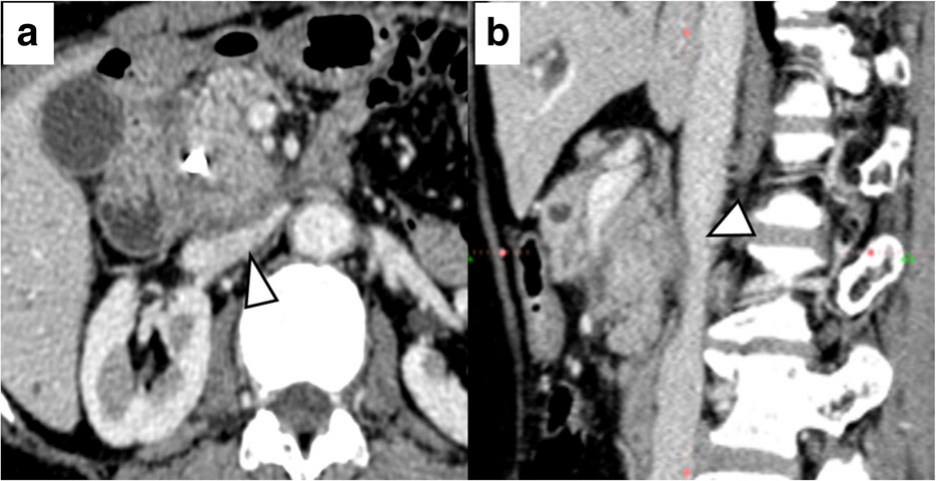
Preoperative computed tomography (CT) imaging of case 3. a Dense soft tissue connected to the pancreatic lesion widely contacting the ventral surface of the inferior vena cava (IVC) (*arrowhead*). b. Sagittal multi-planar reformation showed that the lesion adhered to and deformed the IVC
